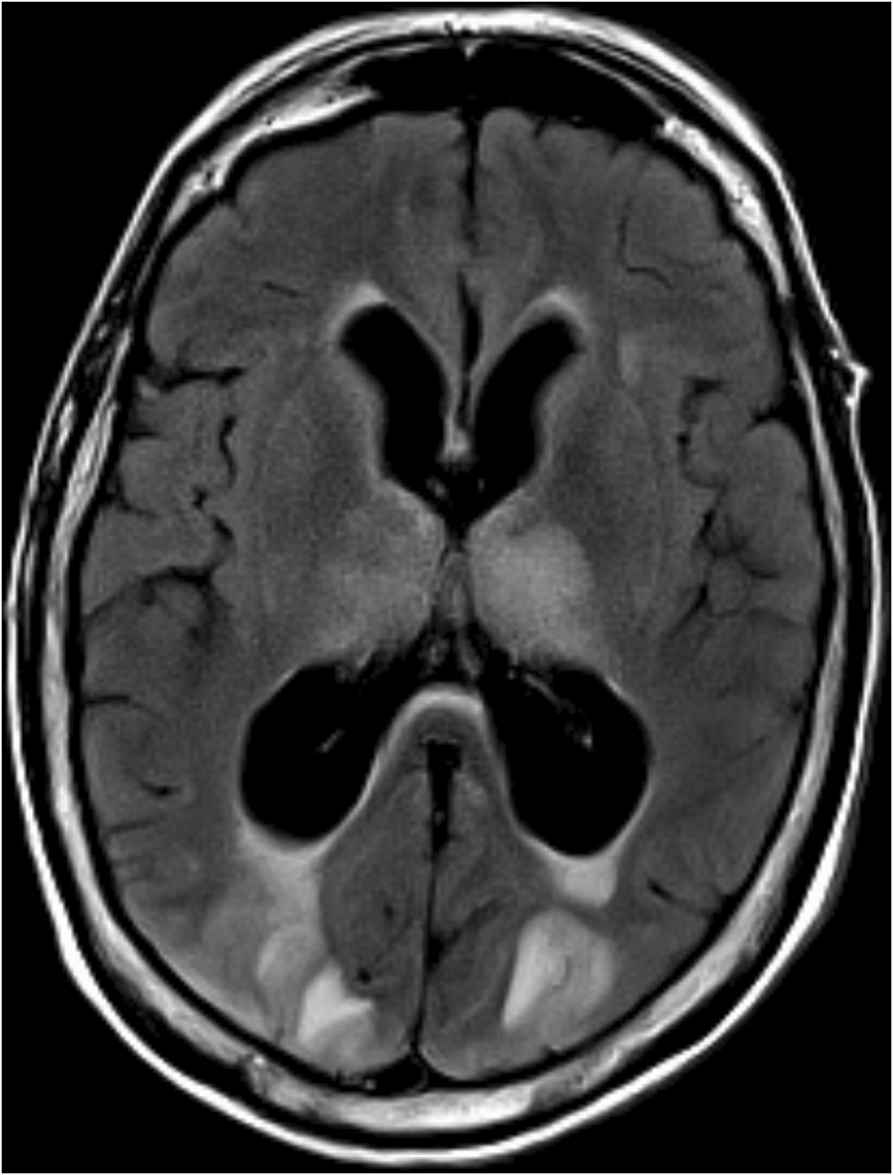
Brain axial fluid-attenuated inversion recovery weighted magnetic resonance image shows hyperintensity in the bioccipital region.
